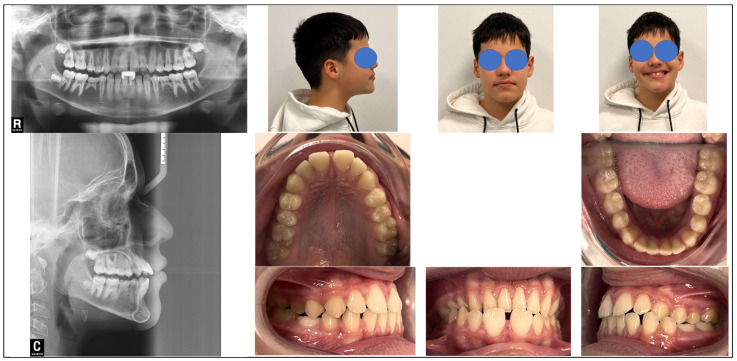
Clinical and radiographic examination. Upper left, panoramic radiograph. Lower left, cephalometric radiograph with Trevisi analysis. Upper right, extraoral pictures. Lower right, intraoral pictures.
